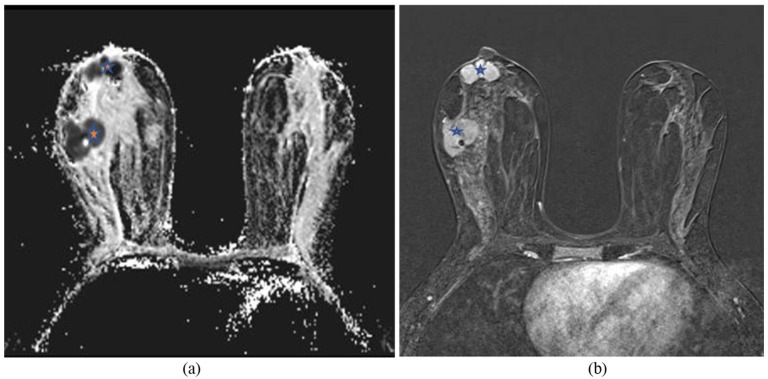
Breast MRI with IV contrast showed a suspicious mass in the right breast with a tissue marker (gel mark) in the dominant mass. (a) Axial ADC image showed hypointense signal masses with low ADC values (0.3; asterisk). (b) T1 Fat-sat subtracted axial image with IV contrast showed an avidly enhancing mass at 9:00 and 9:00 retroareolar extending to the base of the nipple with no signs of nipple invasion (asterisk).
